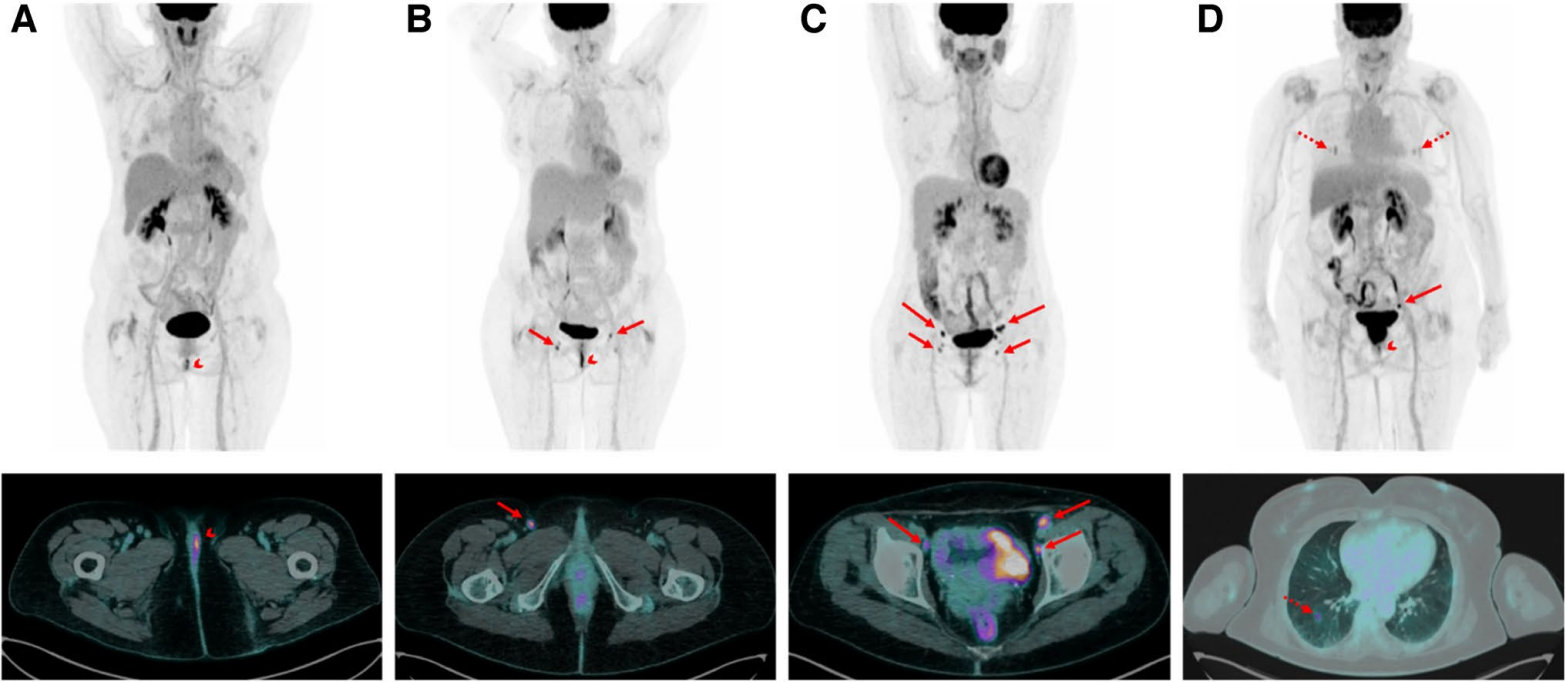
Multiple intensity projection (MIP) (top) and transverse fused PET/CT images (bottom) of four vulvar cancer patients. A A 66-year-old woman with left-side unilateral vulvar squamous cell carcinoma (SCC) of 2.5 cm diameter. PET/CT images showing [^18^F]-FDG uptake just in the primary tumour (arrowhead). This patient was scheduled for partial vulvectomy and ipsilateral SNB. B A 51-year-old woman with midline vulvar SCC of 3 cm diameter. PET/CT images showing [^18^F]-FDG uptake corresponding to the primary tumour (arrowhead) and focal [^18^F]-FDG uptake in bilateral inguinal lymph nodes (short arrows). This patient was scheduled for radical vulvectomy and bilateral inguinal lymphadenectomy. C A 65-year-old woman with midline vulvar SCC of 3 cm diameter previously excised. PET/CT images showing focal [^18^F]-FDG uptake in bilateral inguinal lymph nodes (short arrows) and bilateral pelvic nodes, which were located in the obturator and external iliac regions (long arrows). This patient was scheduled for upfront chemo-radiotherapy. D An 82-year-old woman with vulvar melanoma. MIP showing focal [^18^F]-FDG uptake in the primary vulvar tumour (arrowhead), in the left internal iliac node (long arrow) and bilateral pulmonary nodules (dashed arrows). Transverse fused PET/CT images showing focal [^18^F]-FDG uptake corresponding to a sub-centimetric pulmonary nodule localised in the right inferior lobe. This patient was scheduled for systemic therapy
